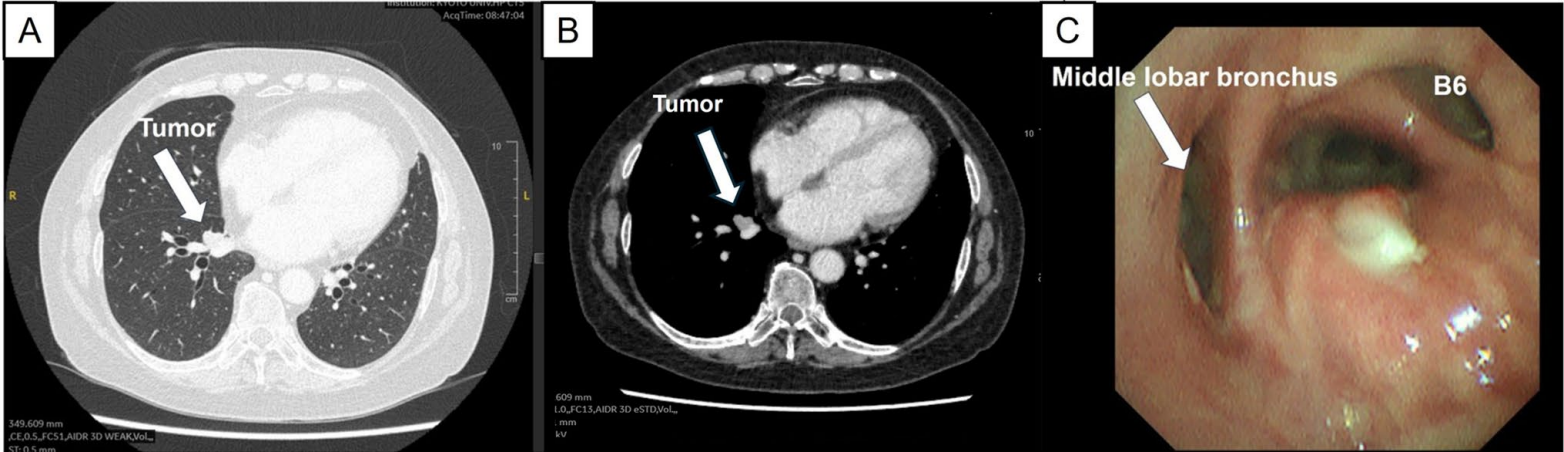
Preoperative findings. A, B Chest computed tomography showed a 1.8-cm tumor in S7. C Bronchoscopy revealed complete occlusion of B7 by the tumor, which had invaded the bronchus intermedius
